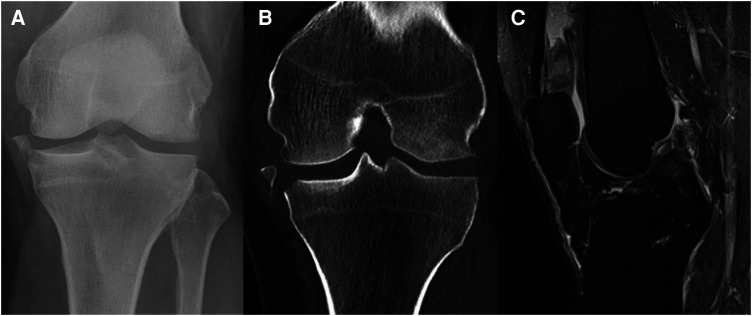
Case 1, 54y male patient, motorcycle accident. AP radiograph (A) and coronal CT reconstruction (B) showing a Moore type III medial avulsion fracture, MRI radiograph (C) showing the concomitant ACL rupture.
